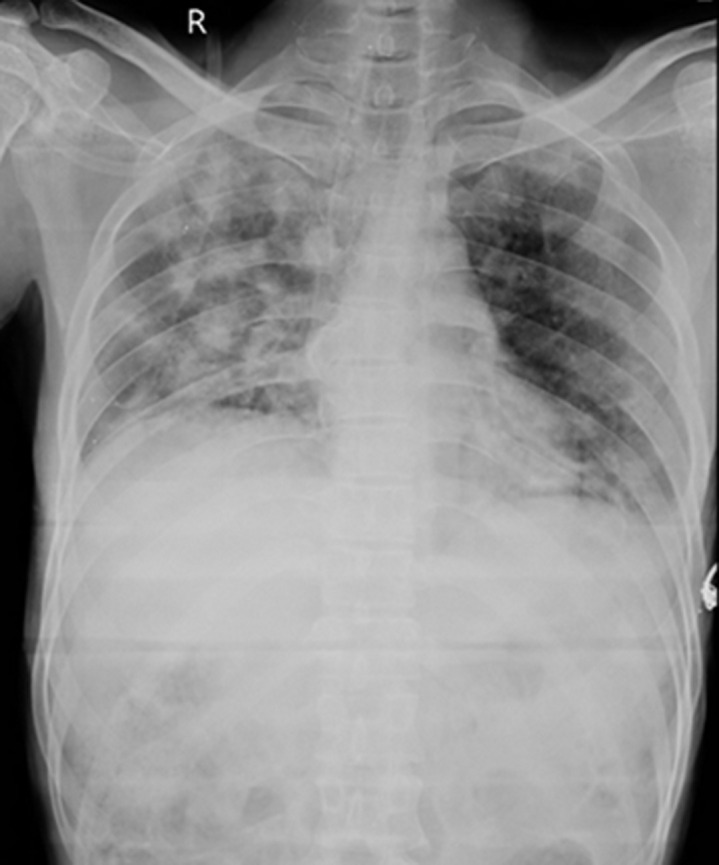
chest X-ray of the patient showing bilateral asymmetrical nonhomogeneous nodular opacities
